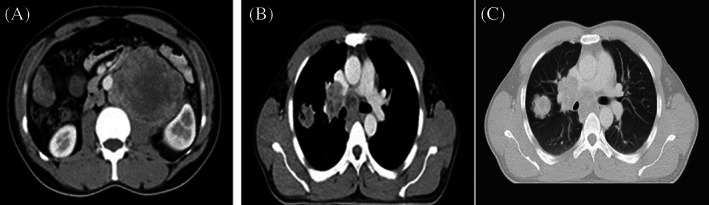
CT scan in September 2019 (at presentation in oncology department). (A) Axial plane of paraaortic lymph node at the level of L2–L3. (B) Axial plane of pathologic hilar and mediastinal lymph nodes at level of T6 (mediastinal window). (C) Axial plane of right pulmonary mass (lung window).
